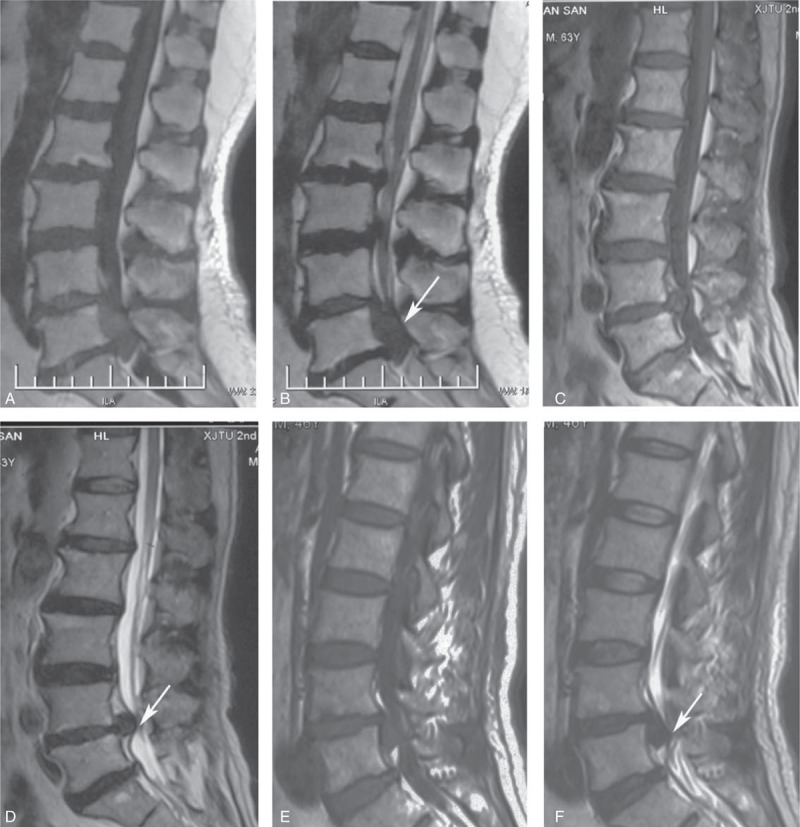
MRI showing the location of the disc fragments. (A, B) Cephalic type. (C, D) Ventral type. (E, F) Caudal type. (A, C, E) T1-weighted images. (B, D, F) T2-weighted images.
